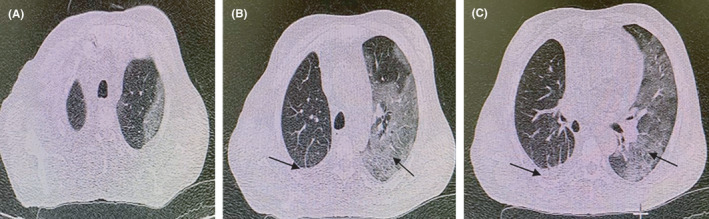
HRCT chest imaging. After four days of treatment. Relative reduction of pleural effusion, collapse of the lower lung on the right hemithorax, and sporadic consolidation and multifocal ground glass opacities on the left lung during management
